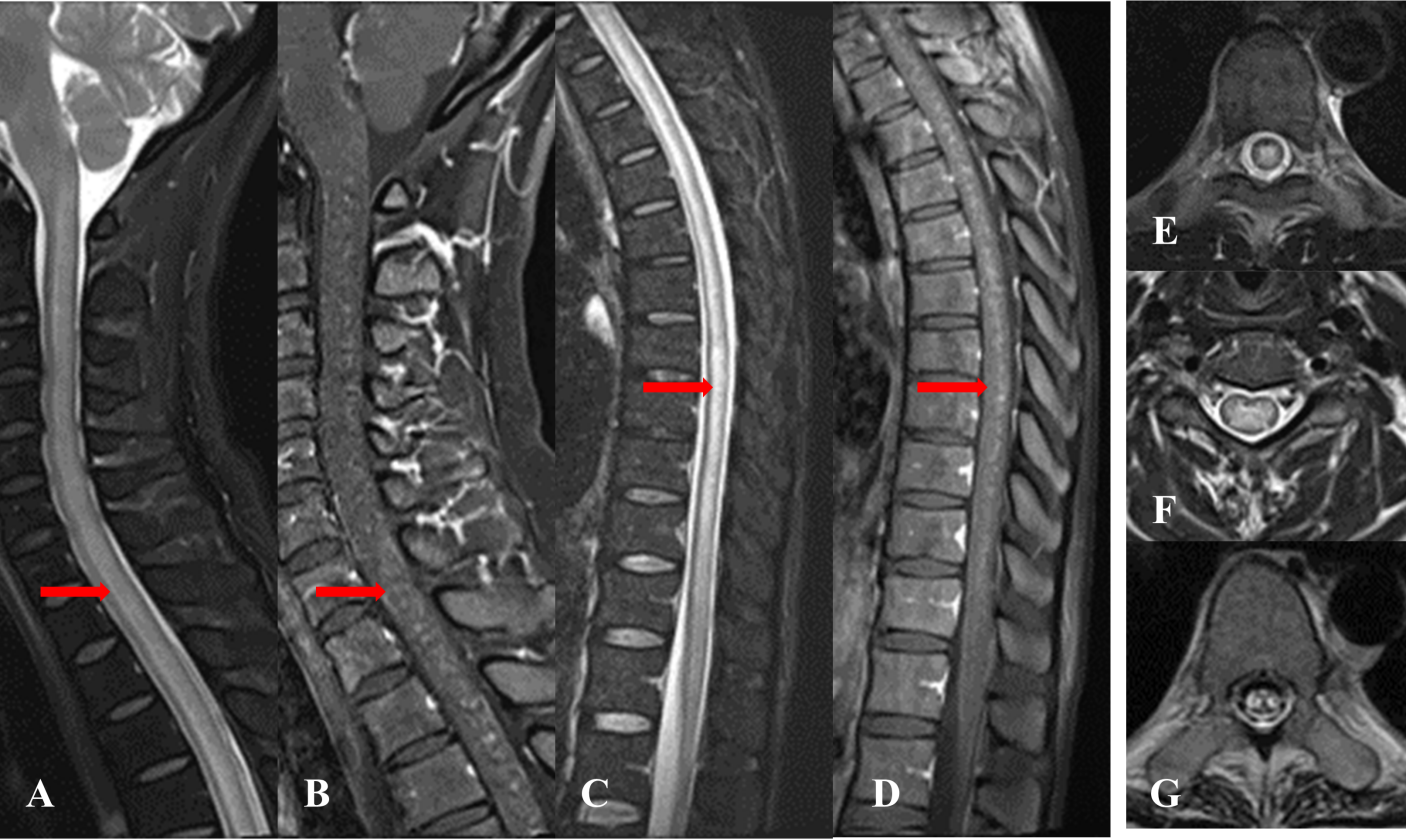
(A, C, E, F) Sagittal and axial T2-Short tau with inversion recovery (T2-STIR) of the cervical and thoracic spinal cord in patient #4, with diffuse central gray matter hyperintensity, (B, D) Sagittal T1 gadolinium contrast enhanced MRI, of the cervical and thoracic spinal cord, patient #4, with patchy focal enhancement in the cervical and thoracic spinal cord. (G) Axial T2-STIR with foci of T2 hyperintensity in the distal thoracic cord, patient #1.
